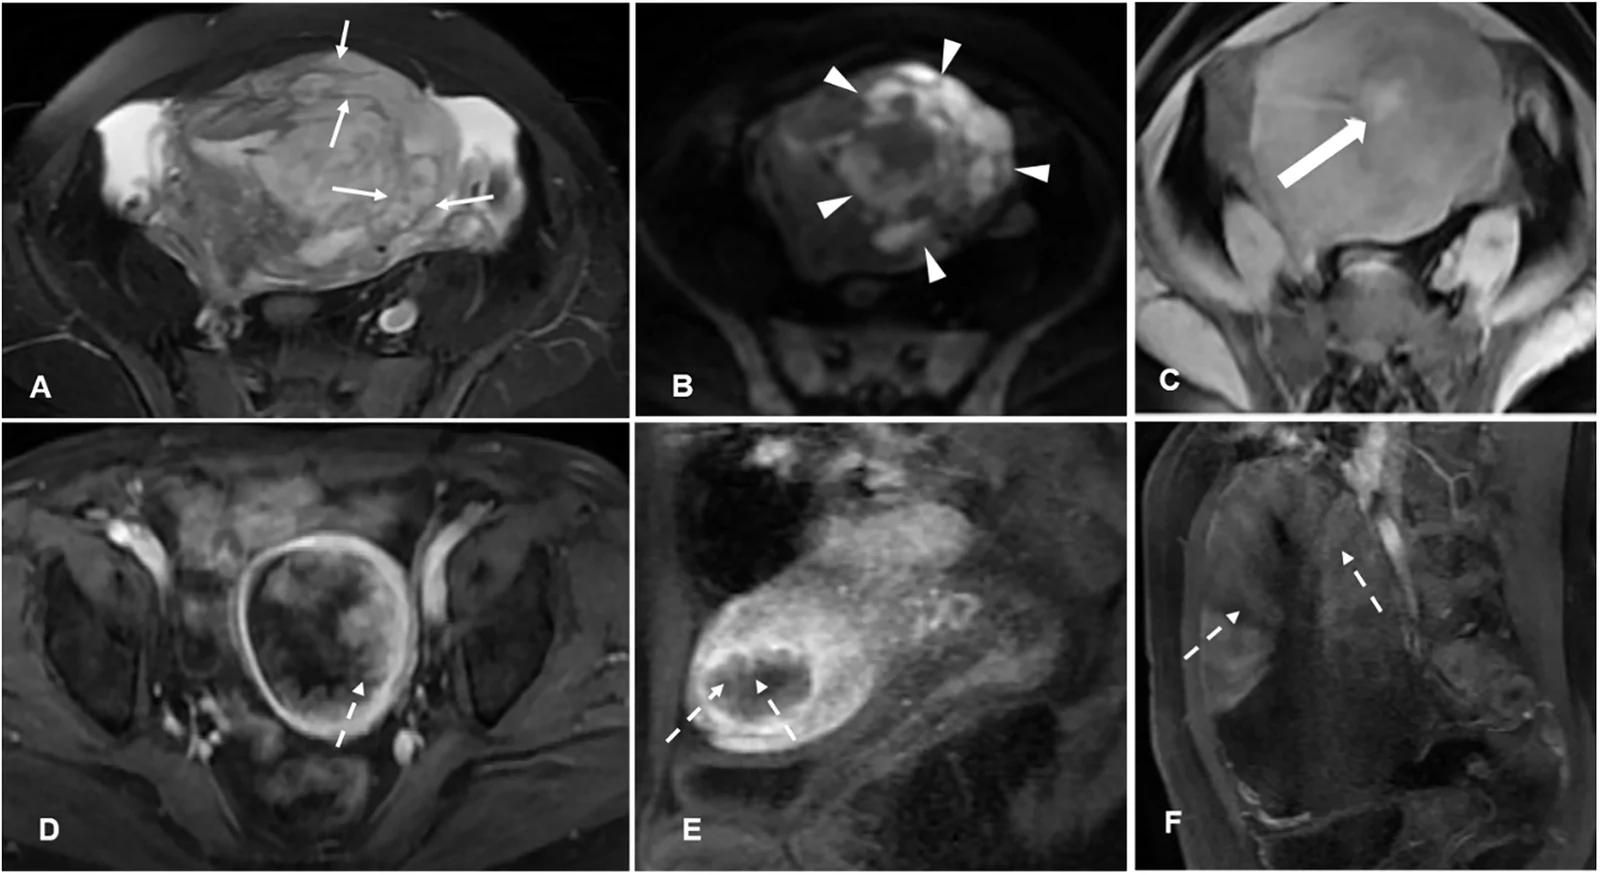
HG-ESS from pelvic MRI of a 49-year-old (A–C), 69-year-old (D), 39-year-old (E), and 37-year-old (F) woman. Axial T2W (A) and DW (B) images showing ill-defined hyperintense lesions within the uterus (arrowheads), containing multiple thin septations and demonstrating a worm-like appearance (arrows). Axial T1-WI (C) revealing hemorrhagic foci within the lesion (broad arrow). Axial (D) and sagittal (E, F) T1-weighted contrast-enhanced images displaying characteristic “feather-like” enhancement of the lesions (dotted arrows)
